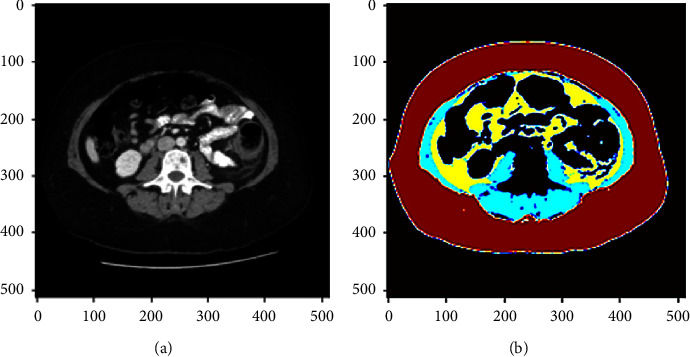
A sample case demonstrating the original CT scan (a) and the AI segmented CT slice (b). In the segmented CT slice, the red indicates the region of SAT, the yellow indicates the region of VAT, and the blue indicates the region of muscles.
